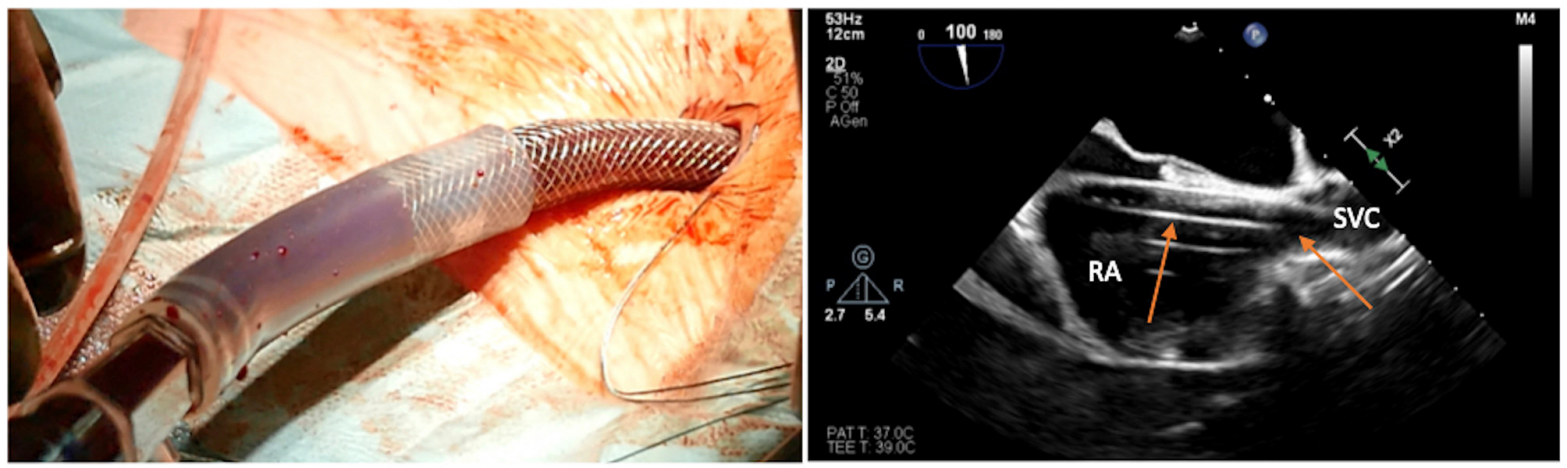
Transesophageal echocardiographic confirmation of positioning in bicaval projection of the tip of the venous cannula in the SVC.
